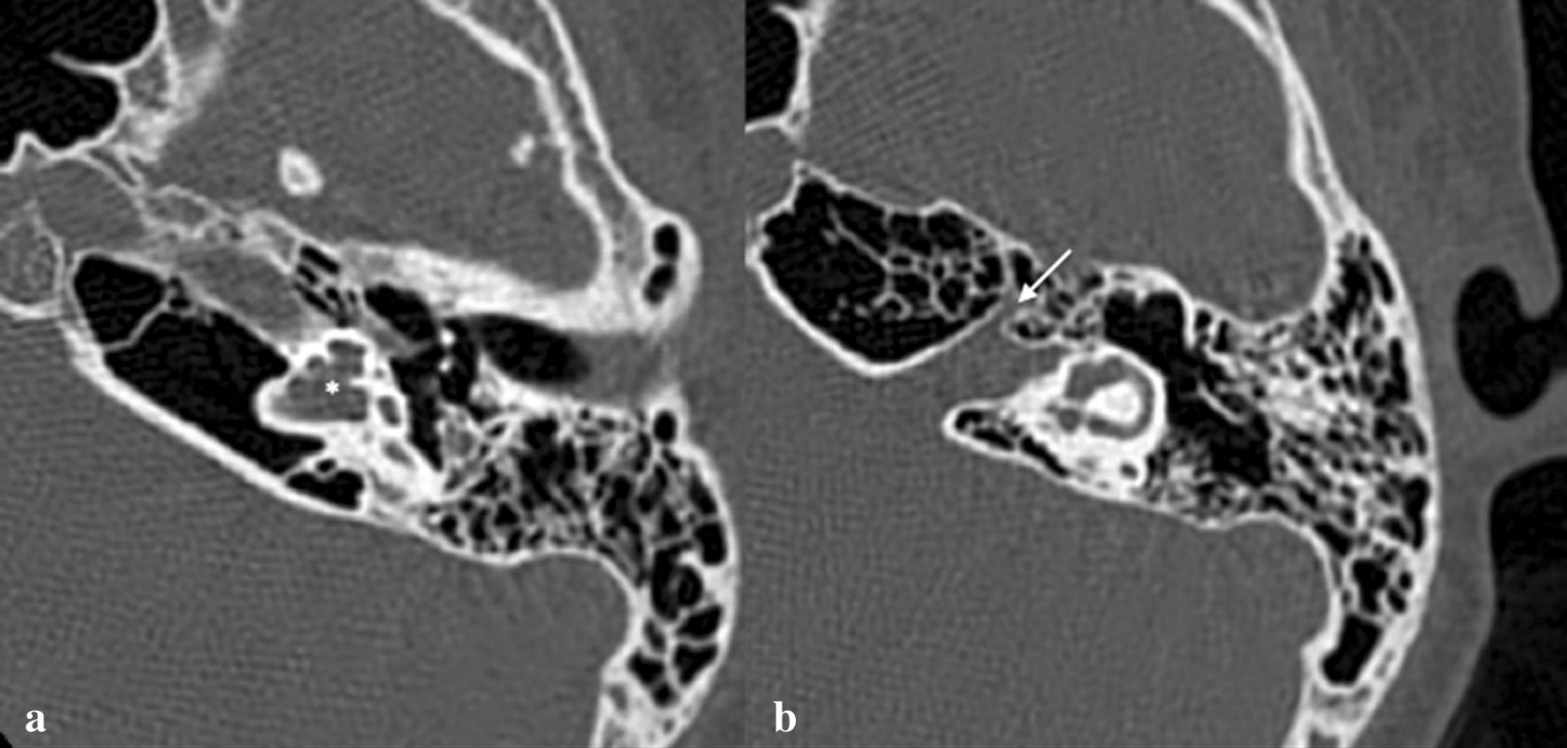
Incomplete partition type-III. Temporal bone axial CT scan of a 12-year-old male with progressive left HL shows an incomplete partition type III (IP-III) (a). The interscalar septa is preserved but no modiolus is appreciable (asterisk in a). There is an assimilation of the cochlea in the bottom part of the IAC with anteromedial dislocation of the labyrinthine segment of the facial nerve canal (arrow in b)
